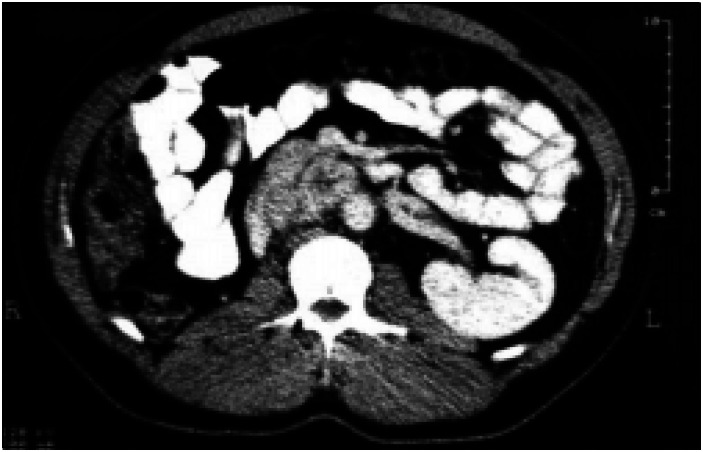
CT scan of the abdomen showing a 3 × 4 cm pre-aortic mass.
